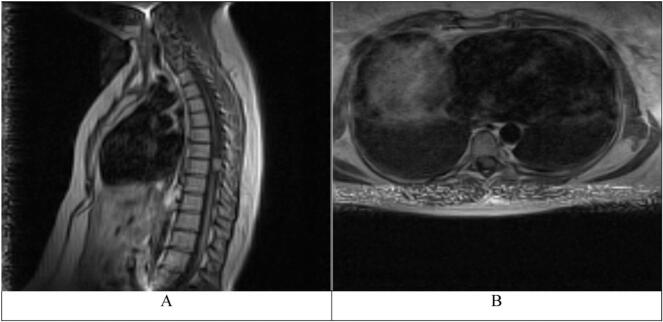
A. T1 weighted MRI, sagittal view. Hyperintense intradural extramedullary mass located at the T8-T9 level. B. T1 weighted MRI, axial view. The mass can be seen compressing the spinal cord anterolaterally.
